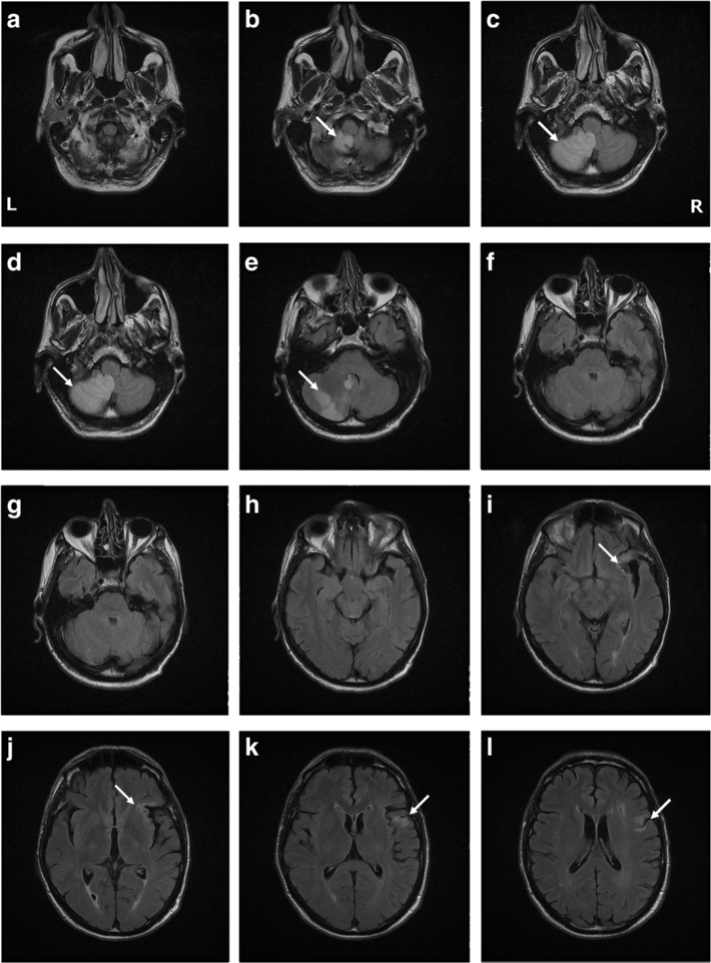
MRI of the cerebellum showing the new cerebellar infarct (a-g) and the old insular lesion (h-l) indicated with arrows
